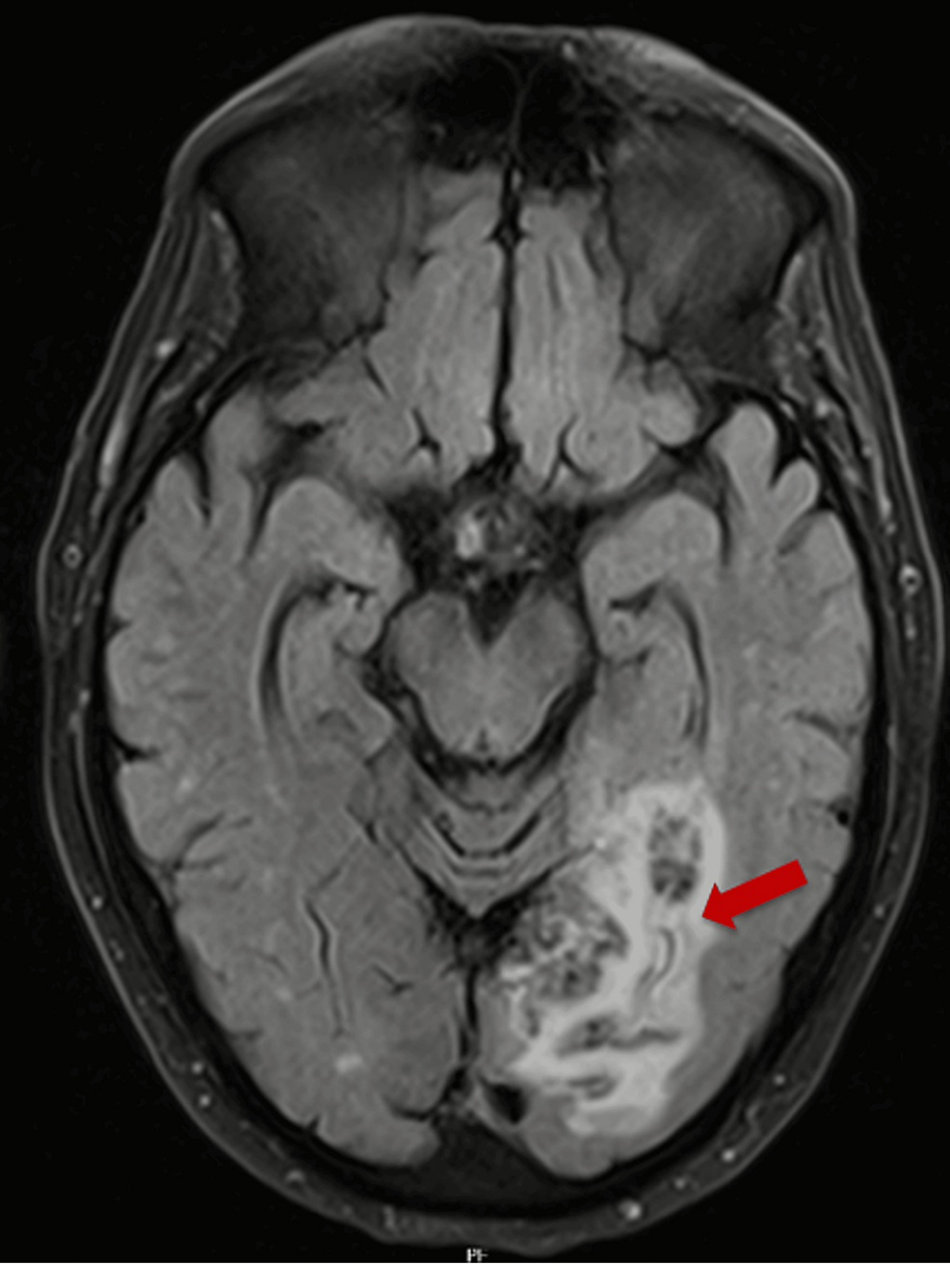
MRI presenting an ischemic lesion with hemorrhagic transformation (indicated by a red arrow)
